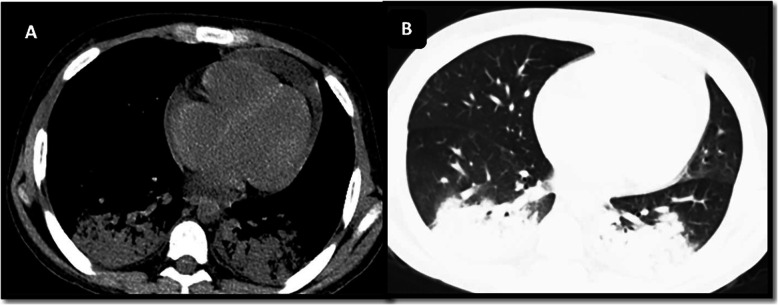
A male patient aged 10 years old with ALL and under chemotherapy developed COVID-19 infection presenting with dyspnea, tachypnea, and fever (moderate clinical presentation). Chest CT revealed pericardial effusion and bilateral basal consolidative patches, with a severity score of 4 (A, mediastinal window; B, lung window)
